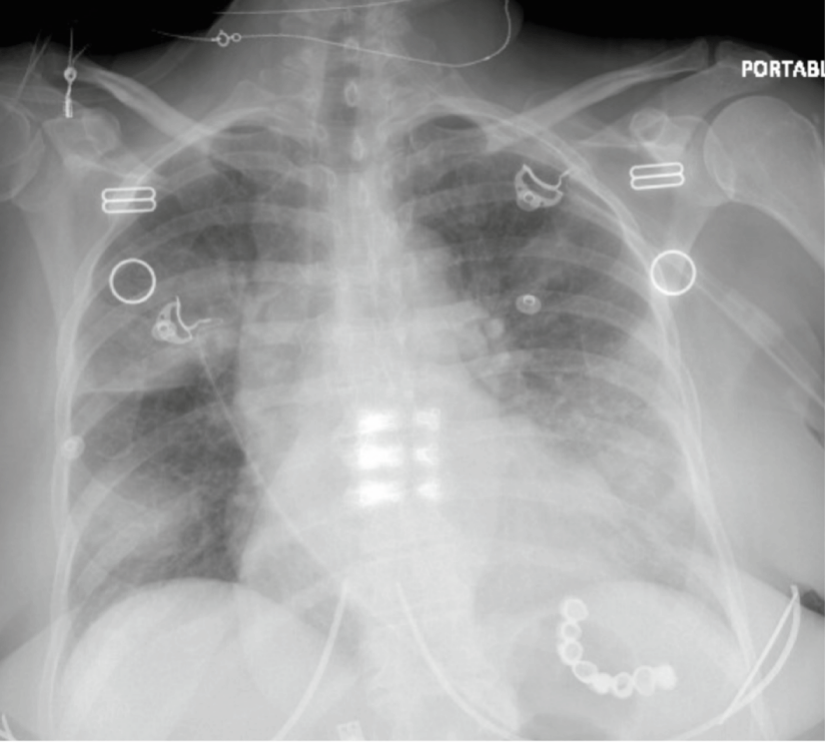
Portable posterior-anterior chest X-ray on admission showing multifocal ill-defined hazy opacities appreciated bilaterally.
